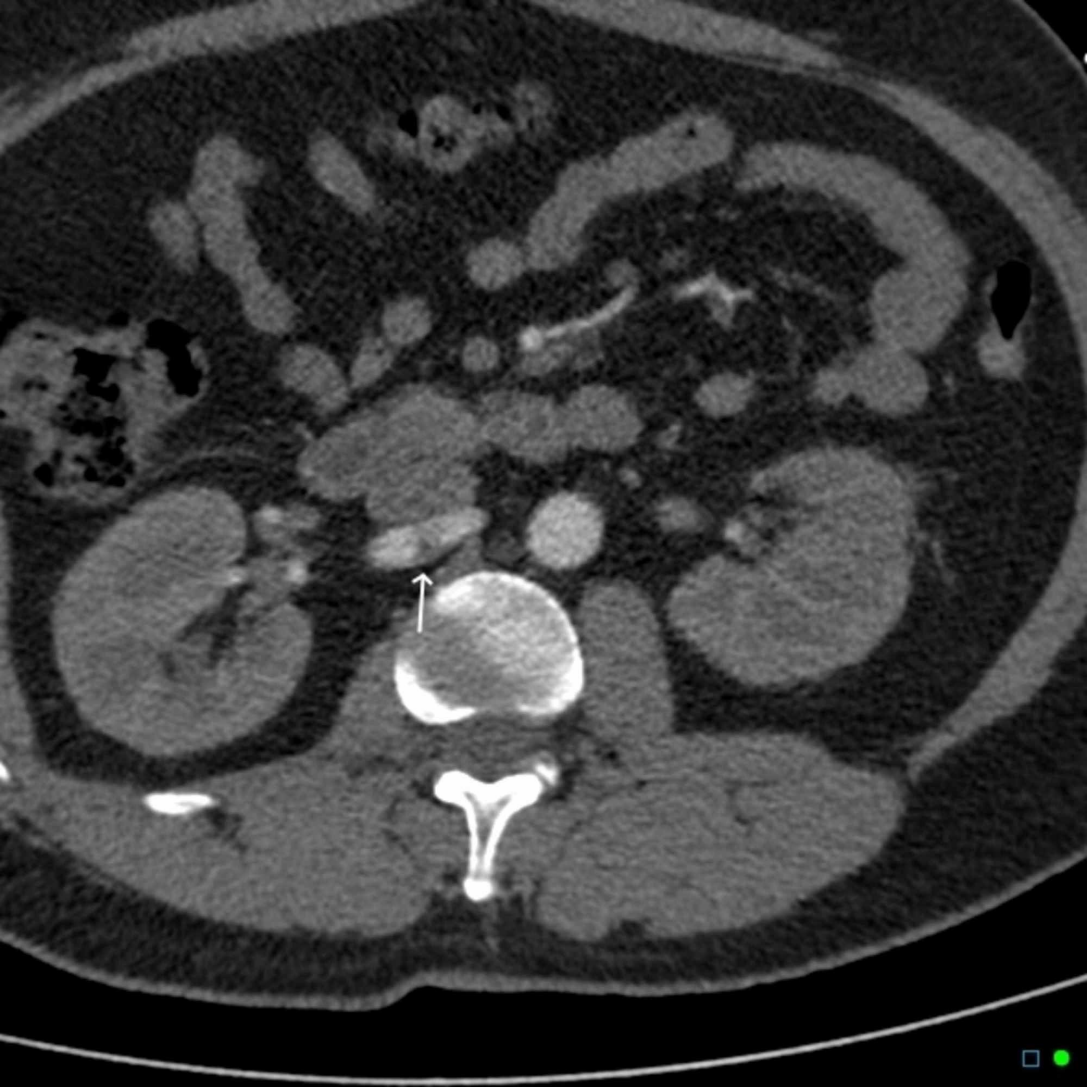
Transverse view computed tomography angiography (CTA) showing right renal artery dissection again - white arrow Figure courtesy: Dr Chris O'Donnell; https://radiopaedia.org/; from the case https://radiopaedia.org/cases/41977
